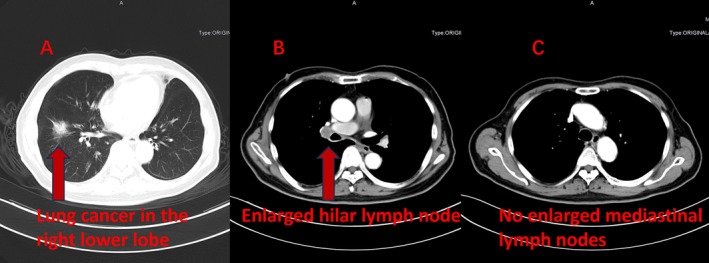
Preoperative computed tomography images of the octogenarian patient. (A) Lung cancer in the right lower lobe. (B) Enlarged lymph node in the hilum. (C) No enlarged lymph nodes in the mediastinum.
